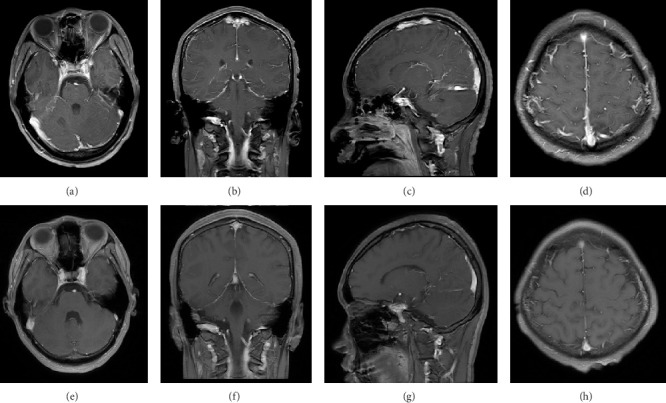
Postcontrast T1-weighted MR of the patient before (a–d) treatment showed diffuse and irregular pachymeningeal enhancement, which attenuated obviously after (e–h) treatment. Multiple dilated dural vessels can be seen between the cranium and brain surface (d), which were resolved after treatment (h).
